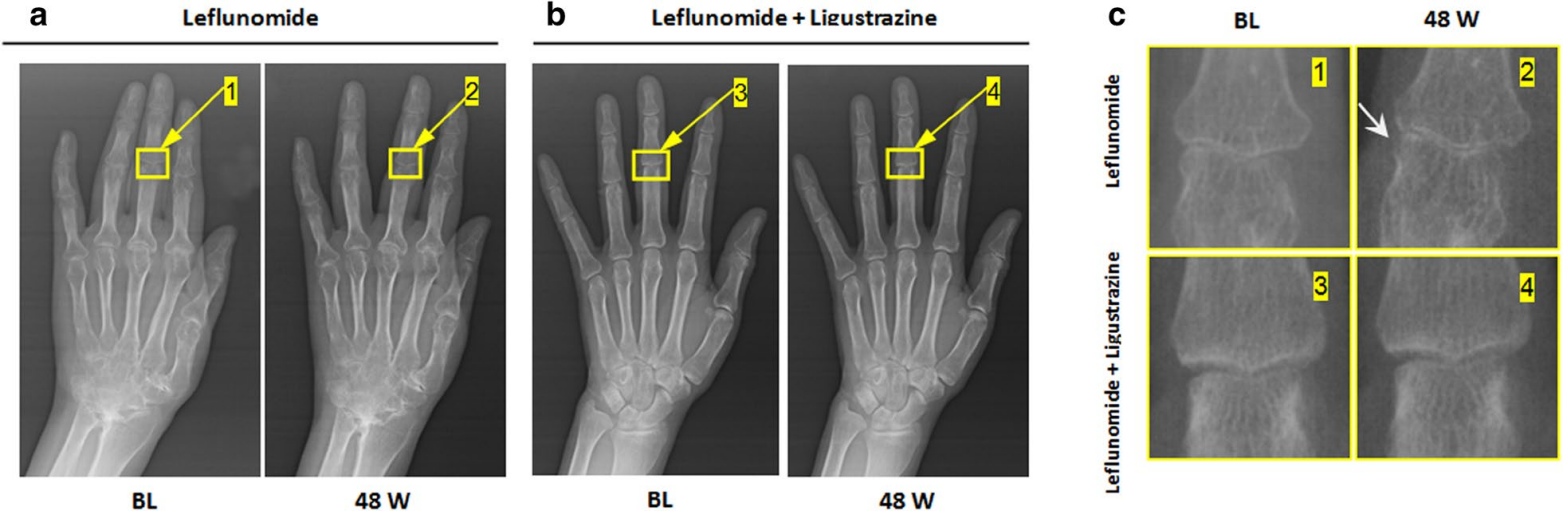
The representative hand X-ray radiographs (left) and enlarged images (right) showing bone cortex erosion (indicated by arrows) at the interphalangeal joint in rheumatoid arthritis (RA) patients before (baseline, BL) and after treatment with either leflunomide (LEF, n = 51) or a combination of leflunomide and ligustrazine (LEF + LIG, n = 61) at week 48, respectively
